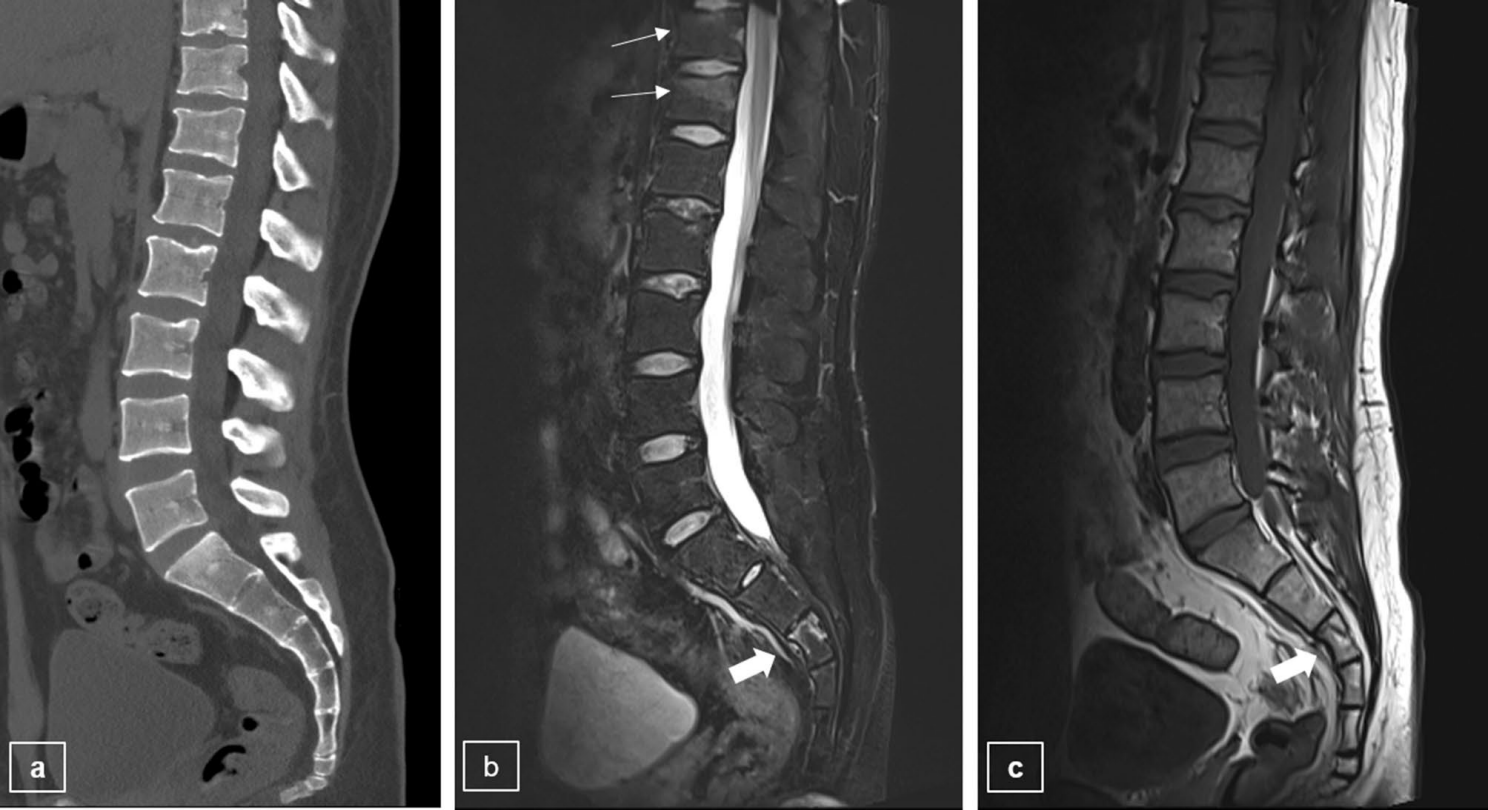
Patients with recent compression fractures at the vertebral bodies of T11 and T12. On modified L-spine CT (a), there is no definite acute fracture at the S3 body, or at T11 and T12. However, on the modified L-spine MRI with T2WI fat saturation (b) and T1WI (c), fractures are shown at the vertebral bodies of T11, T12 (thin arrows), and the upper portion of the S3 body (thick arrow).
